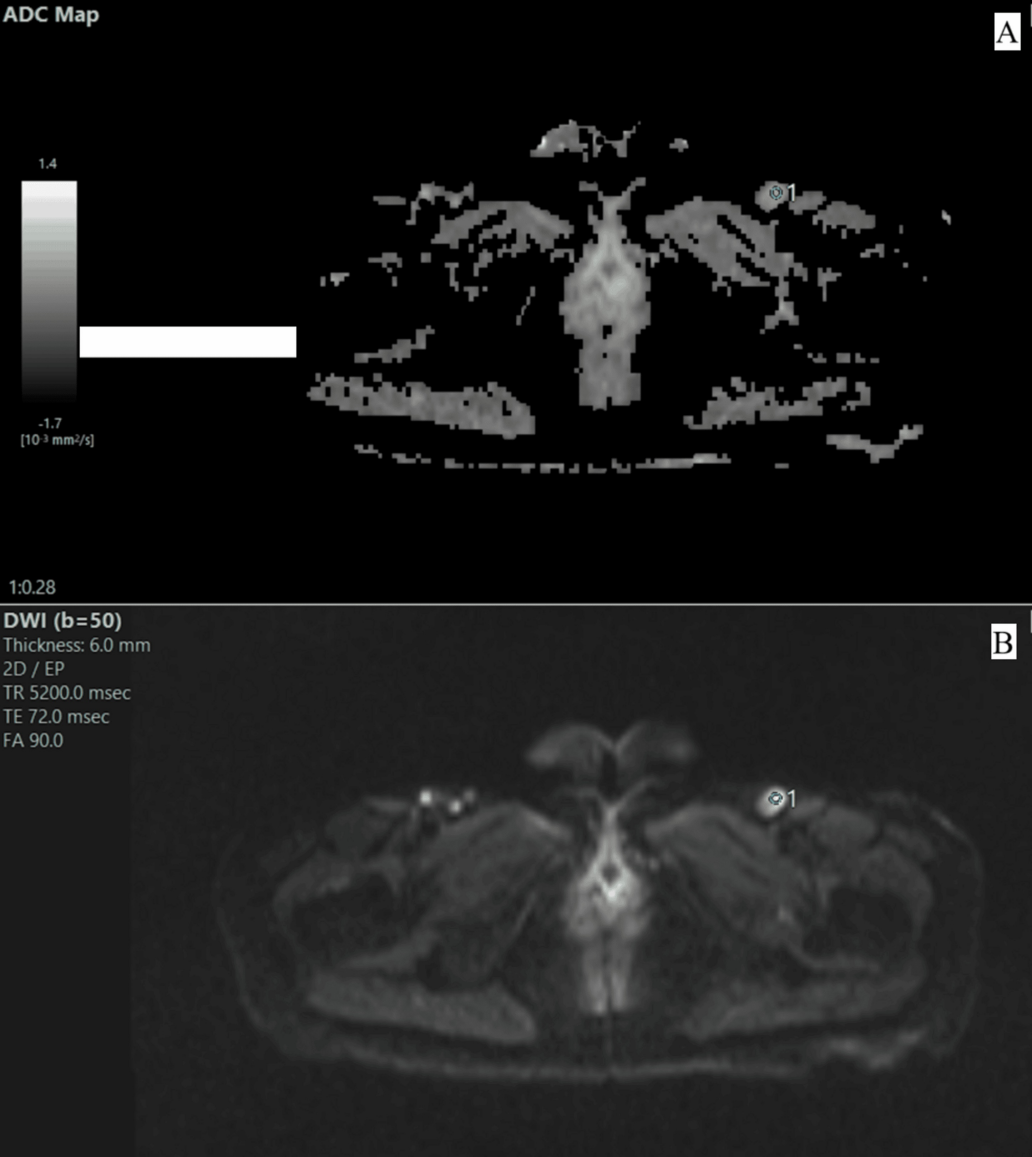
(A and B) DWI and ADC image in which an ROI of 5 pixels is placed on enlarged lymph nodes with a mean ADC of 0.28-suggestive of a suspected metastatic lymph node DWI: diffusion-weighted imaging; ADC: apparent diffusion coefficient; ROI: region of interest
